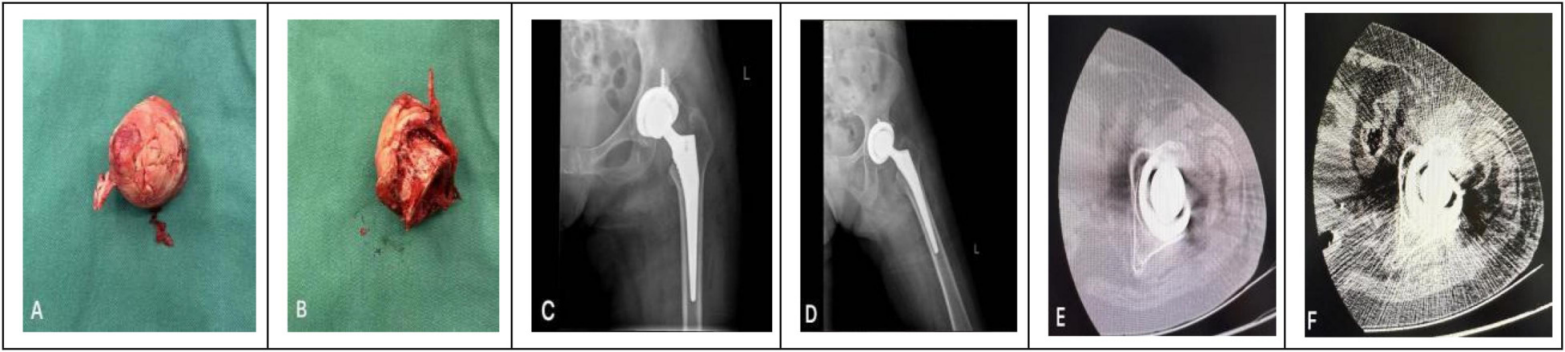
Intraoperative and early postoperative images. (A,B) Necrotic femoral head removed during surgery (arrow indicates collapse and deformity); (C,D) left hip anteroposterior x-ray at 4 days postoperatively (arrow indicates well-aligned acetabular cup and femoral stem, no loosening). (E,F) Postoperative 3-month imaging of the left hip.
